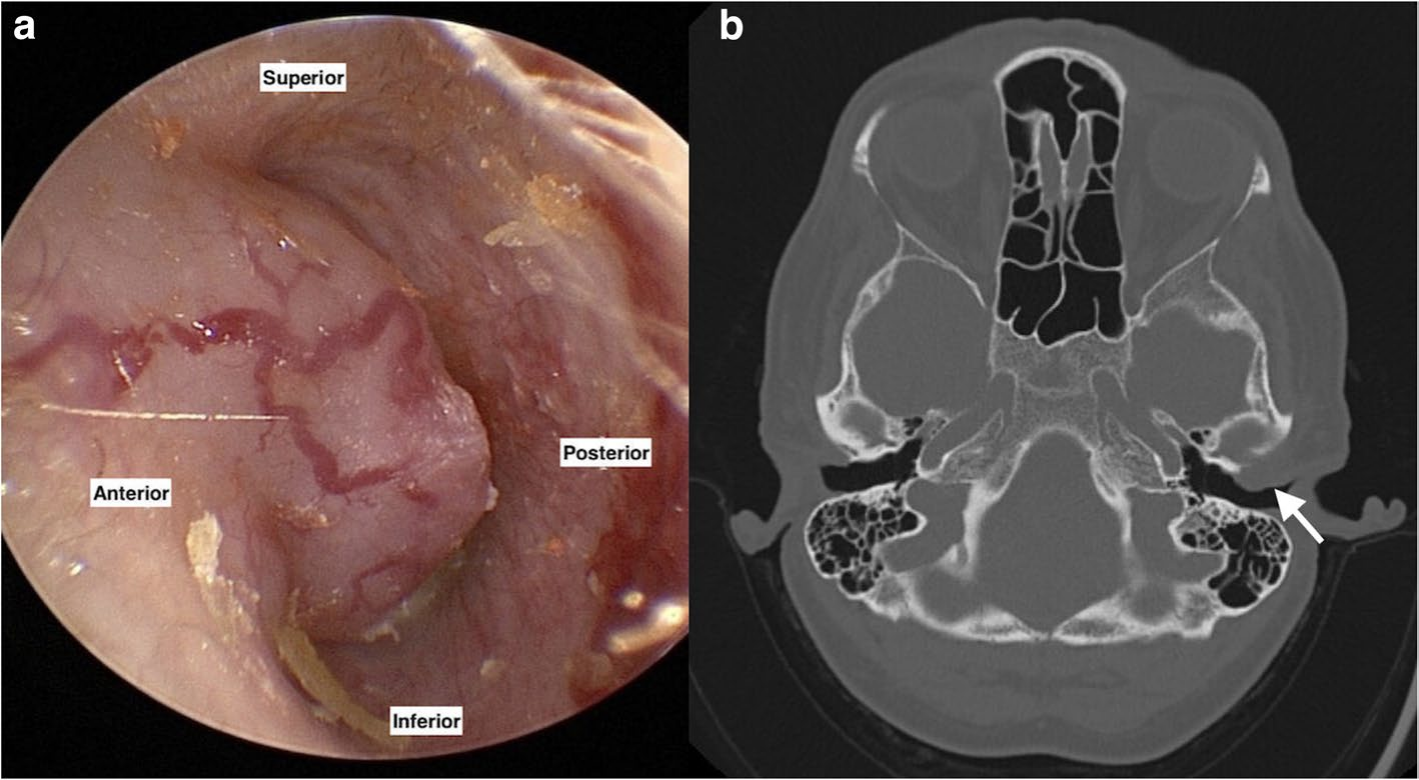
A The endoscopic view of the left ear canal with myoepithelial tumor, anatomical orientation marked (left image). B The preoperative CT scan showing the pathological formation (20 × 11 mm) in the anterior EAC wall (arrow), with the marginal osteolytic process (right image)
